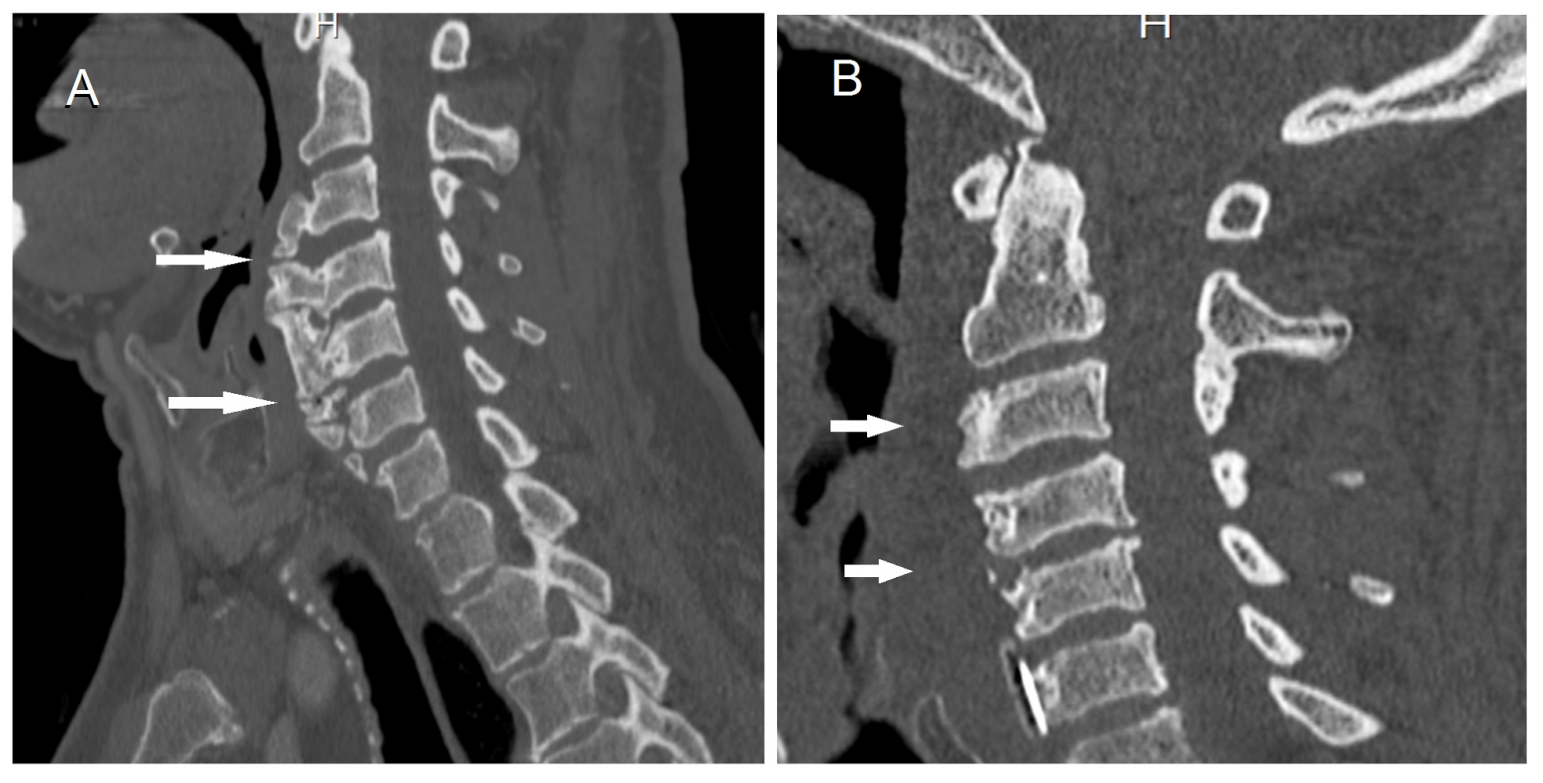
Sagittal cervical computed tomography (CT) scan. Arrows point to comparable regions. A) Preoperative sagittal cervical CT scan showing anterior hyperostosis. B) Post-osteophytectomy cervical CT scan
